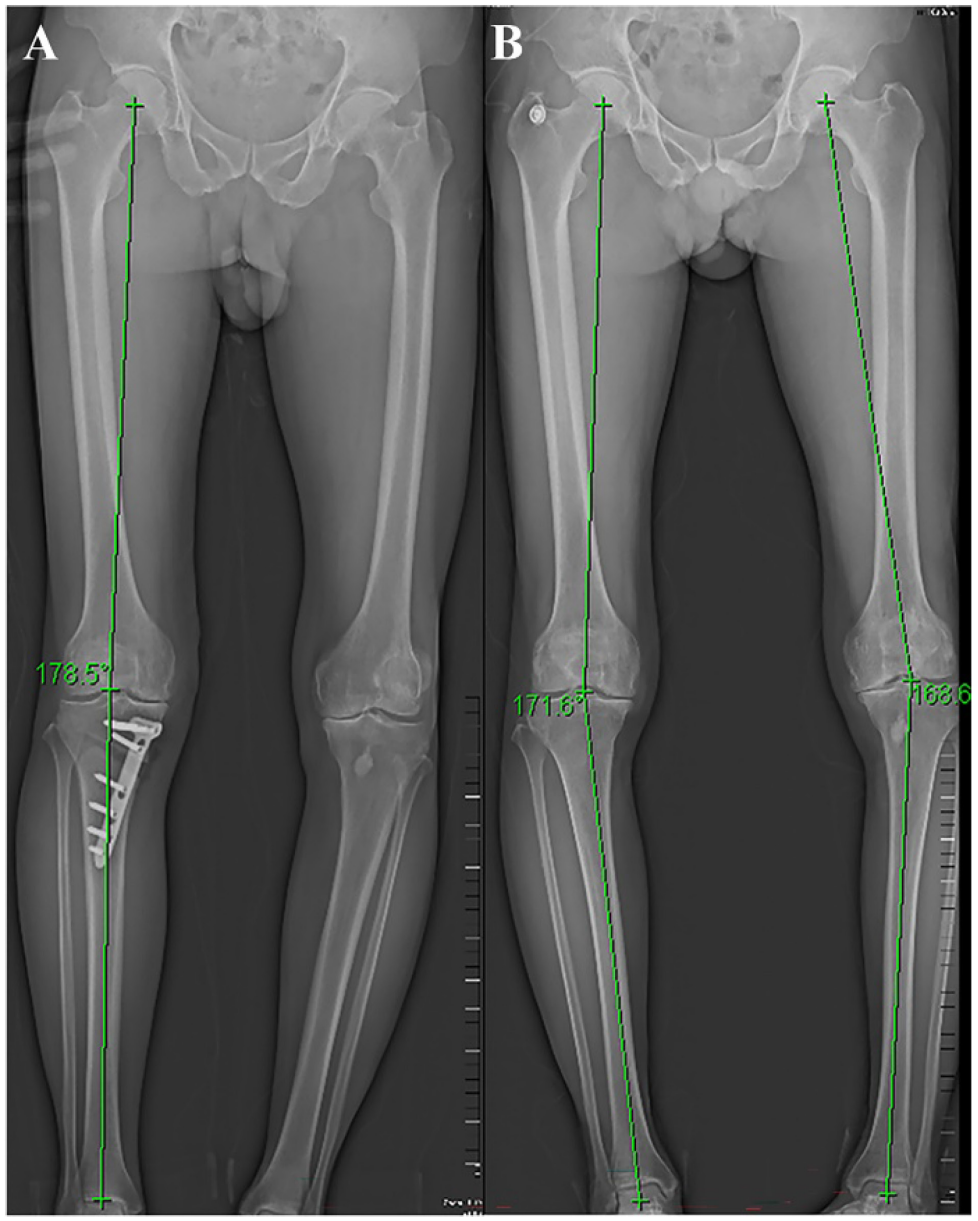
Postoperative (A) and preoperative (B) lower limb X-ray and force line.
